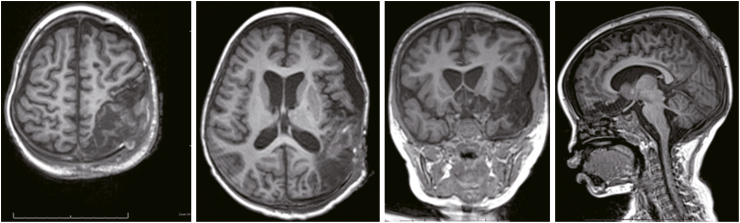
MRI showing a large multi-territorial infarct involving the majority of the left cerebral hemisphere and medial aspect of the right frontal lobe with established encephalomalacia and atrophy. There are blood products of different ages in the left parietal lobe.
